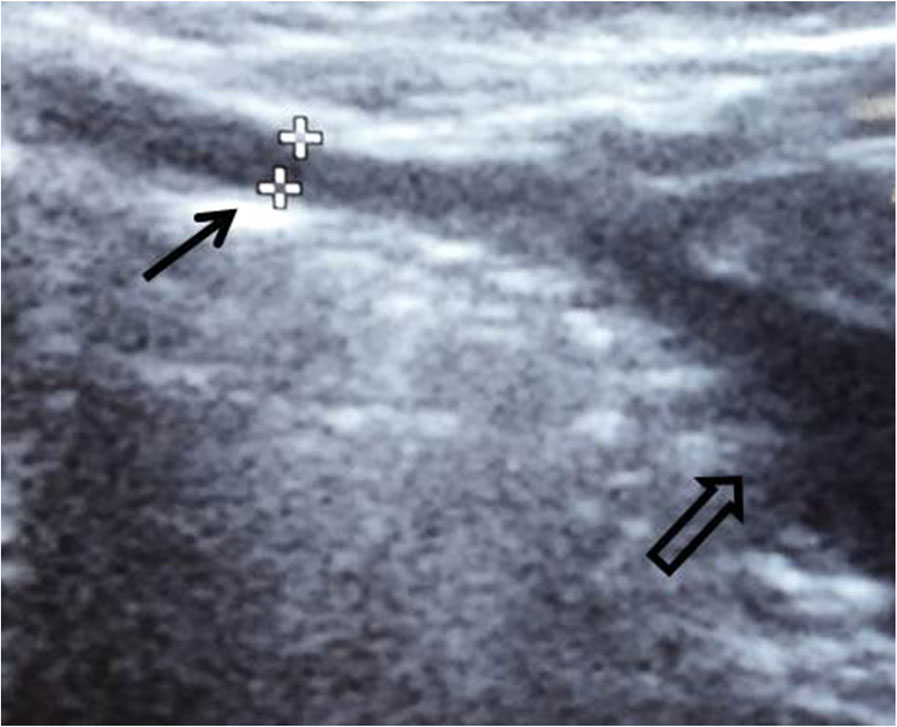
Ultrasound findings. There was a tubular structure (*black arrow*) traced from umbilicus with possible connection to the urinary bladder (*hollow arrow*)
